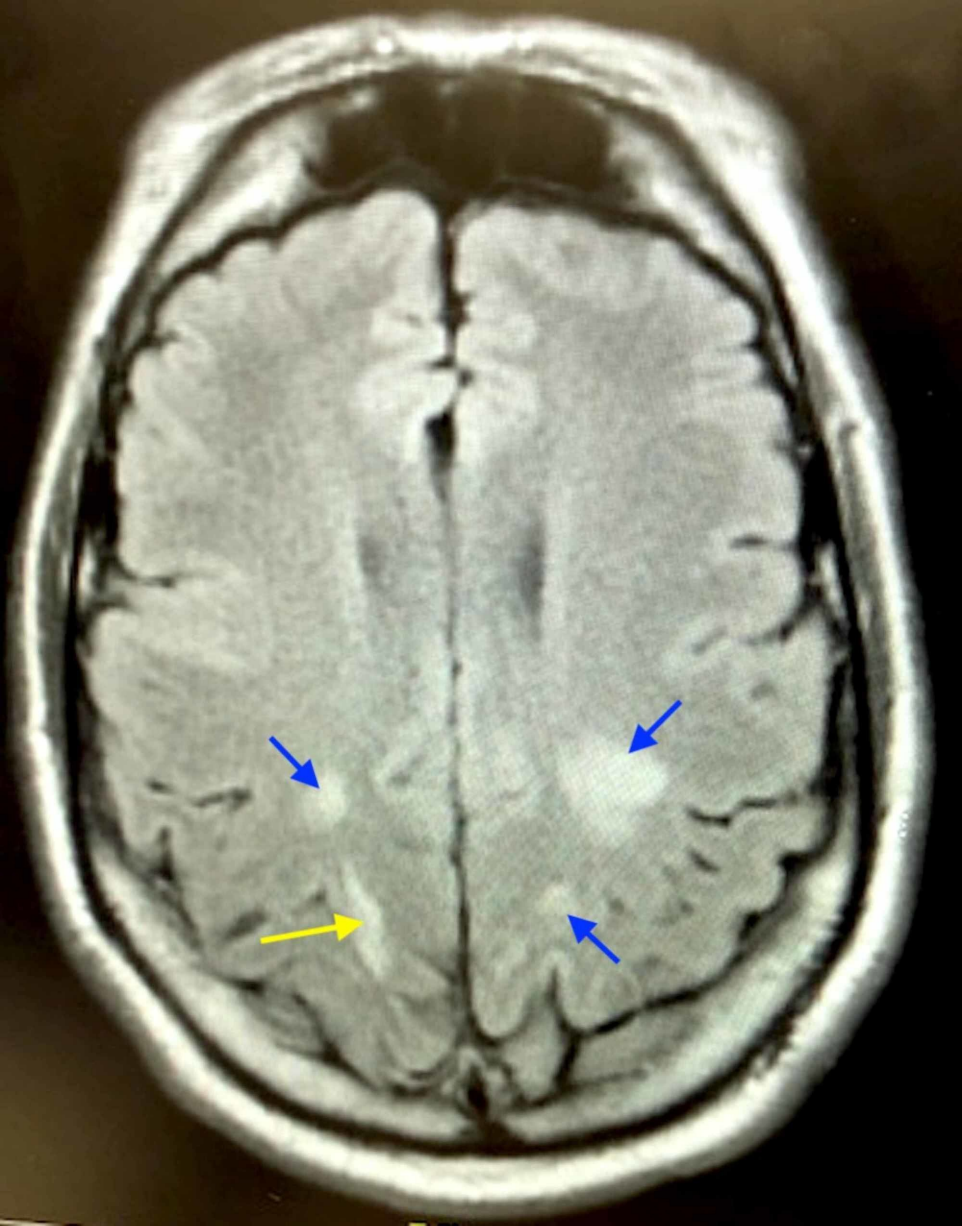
MRI demonstrating bilateral parietal and occipital subcortical T2 hyperintensities (blue arrows) with right occipital artifactual restricted diffusion (yellow arrow). No evidence of acute infarct, hemorrhage, hydrocephalus, or mass.
